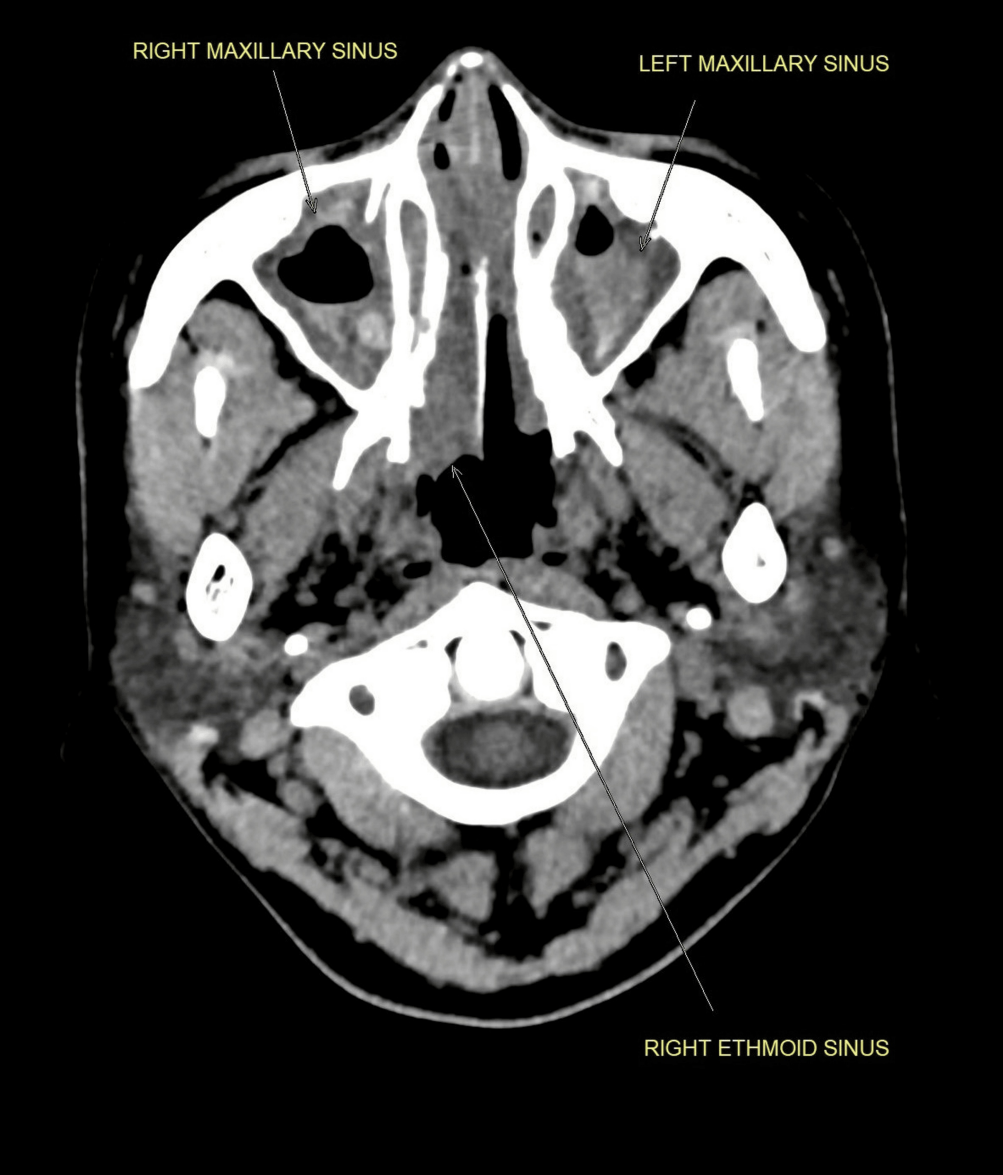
Non-contrast computed tomography of the head shows mixed iso-dense and hyperdense soft tissue material filling up the ethmoid and bilateral maxillary sinuses (yellow arrow)
